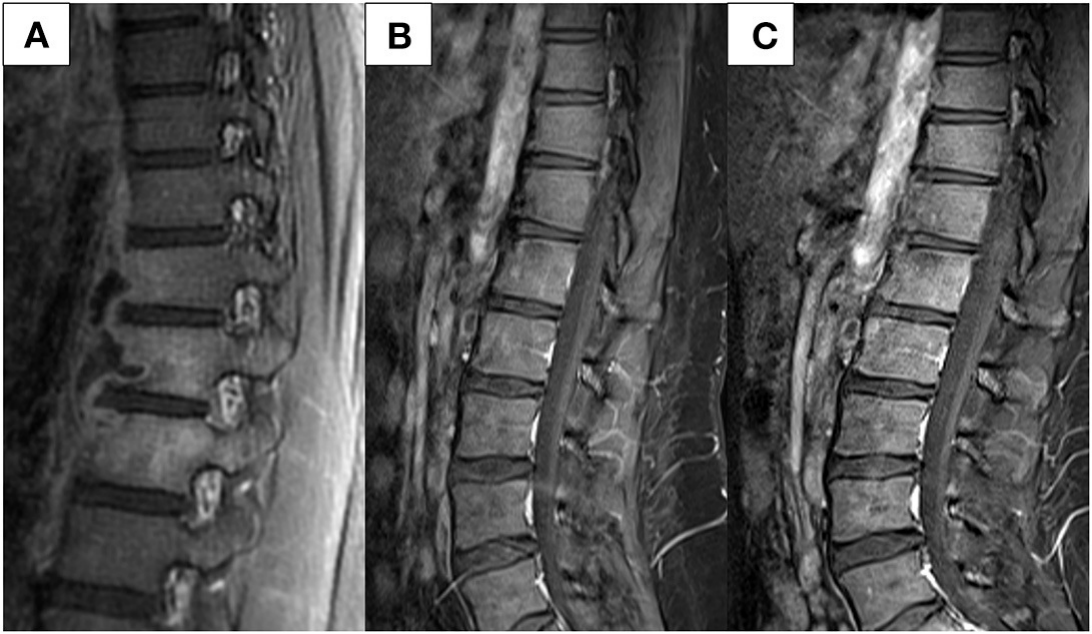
Spinal MRI conducted (A) at diagnosis of spinal TB and (B,C) follow-up images. (A) Bone marrow enhancement at T11-12-L1 with paraspinal fluid collection at left anterolateral side, and relatively thin wall enhancement. (B) Improvement is noted at 1-year follow-up after TB treatment initiation. (C) Imaging conducted 1 year after VDZ initiation shows no reactivation of the TB spondylitis. MRI, magnetic resonance imaging; TB, tuberculosis; VDZ, vedolizumab.
